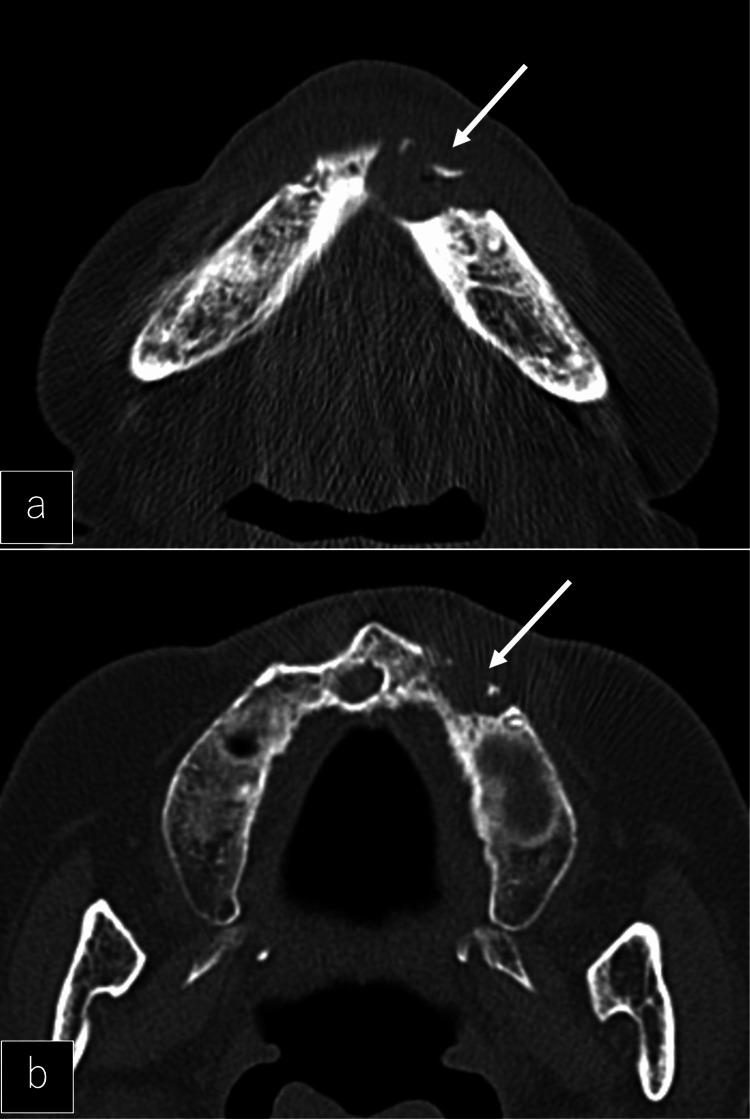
Necrosis of the jaw on CT a: Axial CT showed a bone translucency spot on the left mandible (white arrow). b: Axial CT showed a bone translucency spot on the left maxilla (white arrow).
